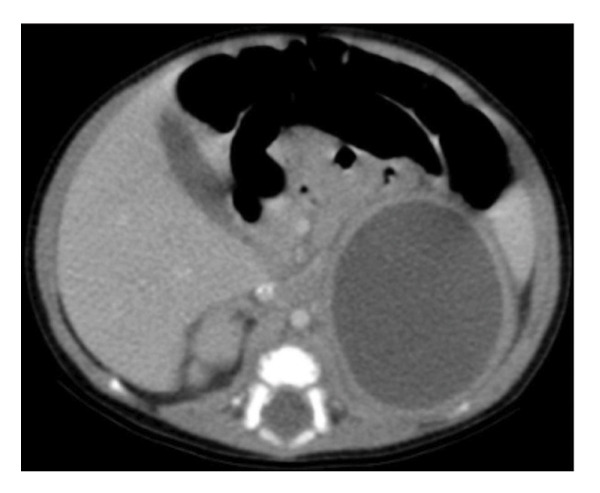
Contrast‐enhanced CT scan (Day 20) showing a left adrenal rim‐enhancing cystic mass without calcification or solid component, consistent with adrenal hemorrhage.
